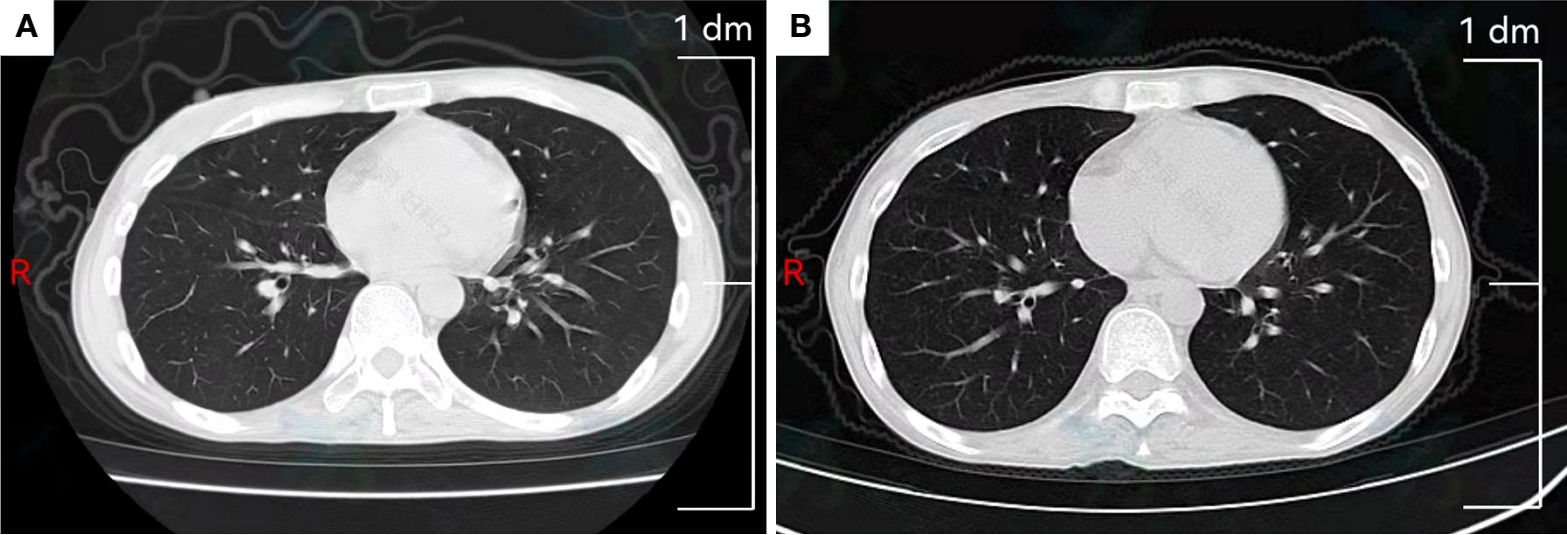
Chest computed tomography scan. (A) Slight interstitial changes in the lung before BEP´ chemotherapy. (B) Slight interstitial changes in the lung after BEP´ chemotherapy.
